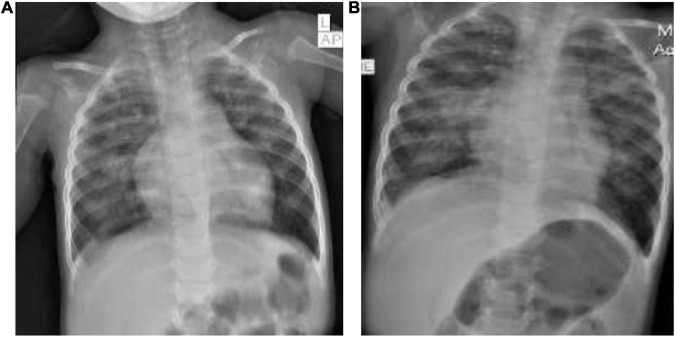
(A,B) Chest x-rays of the third patient.
